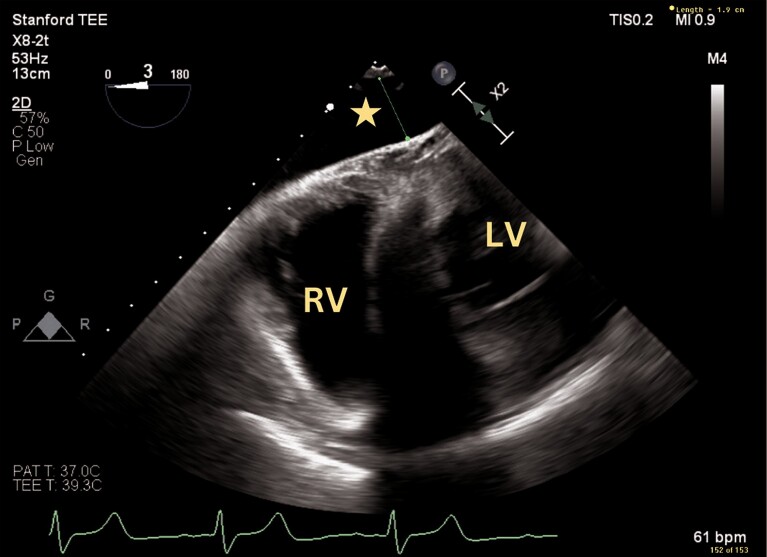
Identification of pericardial effusion (star) in gastric transoesophageal echocardiogram view. LV, left ventricle; RV, right ventricle.
